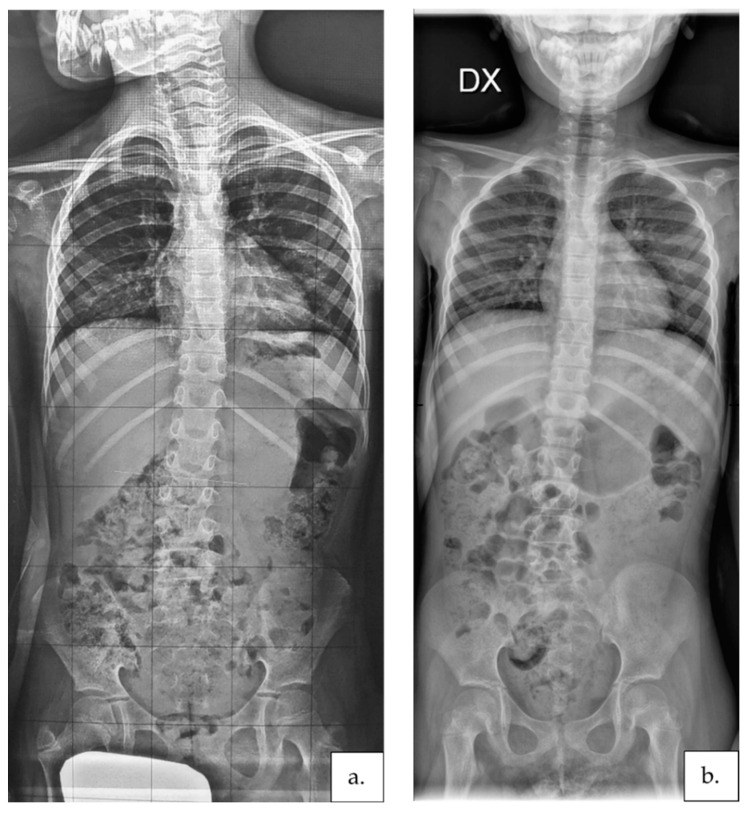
Participant no. 8′s X-rays collected before (a) and after (b) the intervention. She was 6.5 years old at T1. At baseline, she could walk for more than 10 steps supported by one hand and independently stand for 30 s. Lack of efficient balance reaction was recognized at T1. Therefore, her program included intensive balance training and asymmetrical posture maintenance. At the end of the intervention, she could walk for more than 10 steps and stand for more than one minute independently. An improvement of 10° Cobb was recognized at T2.
